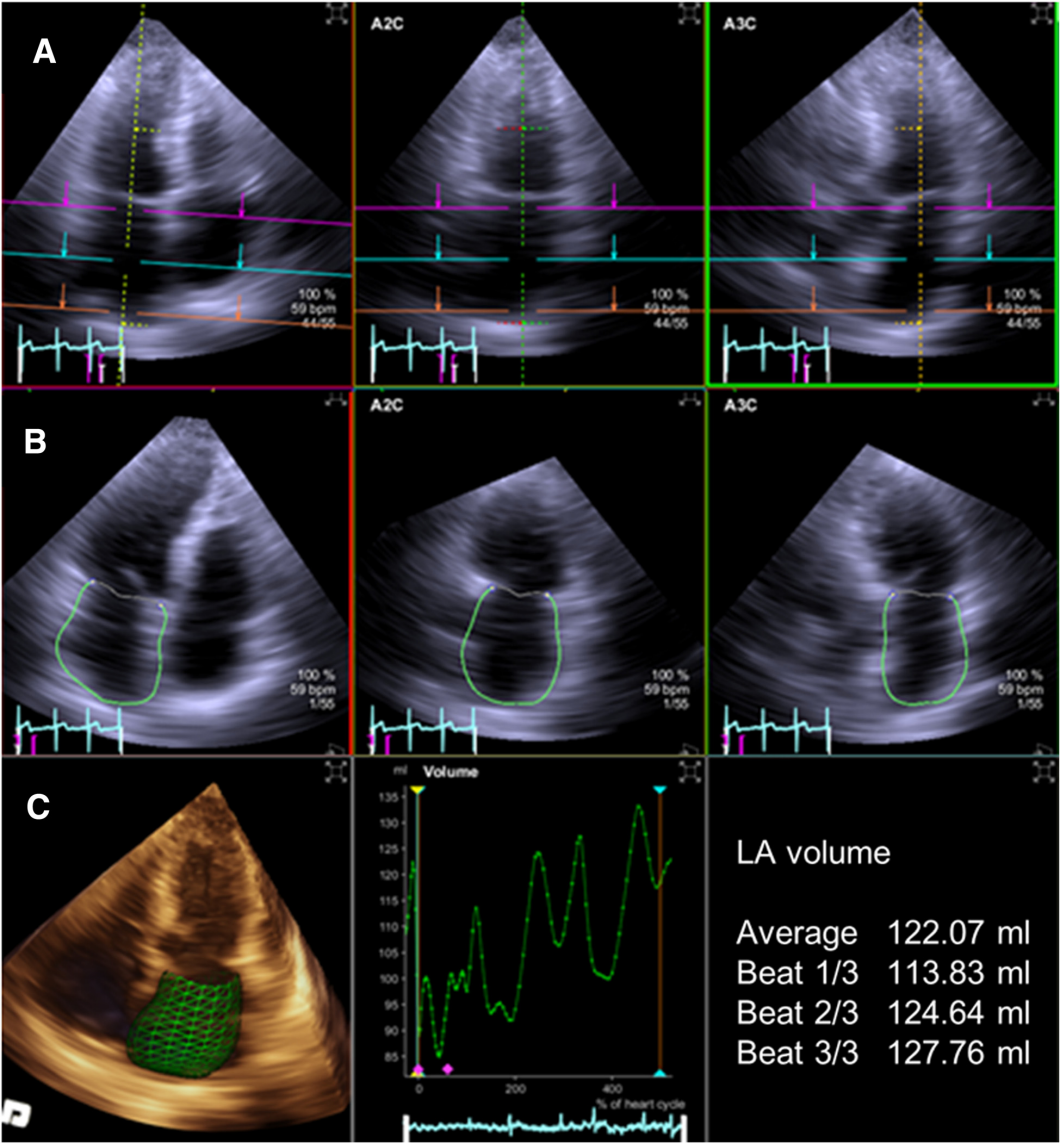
Measurement of left atrial volume by three-beat averaging real-time three-dimensional echocardiography with semi-automated endocardial border detection. a Set the region of interest in the left atrium. b Perform semi-automated endocardial border tracking of the left atrium. c Set left atrial volume curve, and calculate average left atrial LA volume from three consecutive beats
